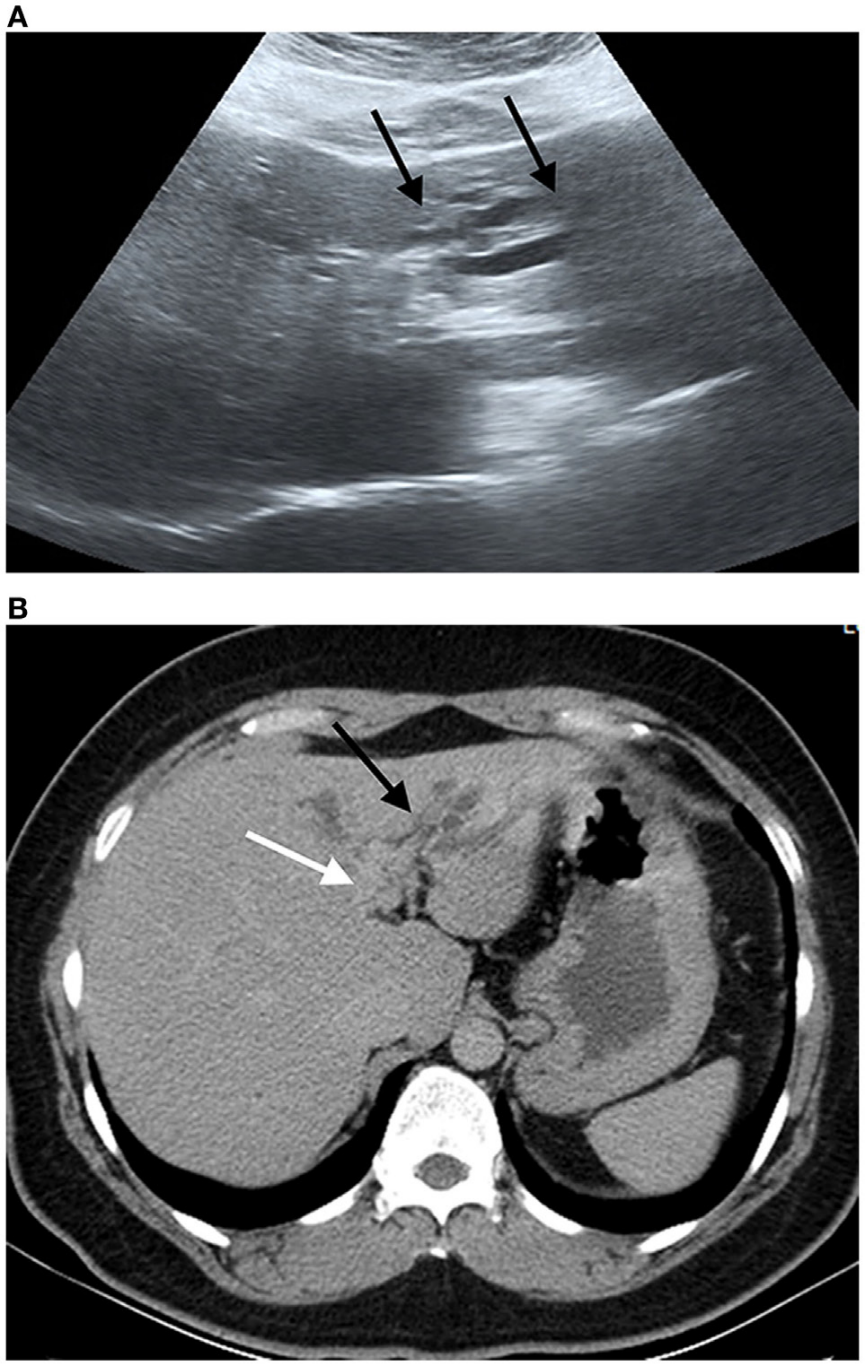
Ultrasound image and compute tomography in cholangiocarcinoma (CCA). (A) Ultrasound image shows a mild degree of dilatation of the intrahepatic bile duct left lobe of the liver (black arrows). (B) Portal phase computed tomography shows infiltrative tumor along left hepatic duct (white arrow) with dilatation of intrahepatic duct in left lobe (black arrow). Final diagnosis is perihilar periductal CCA stage II (AJCC 7th edition).
